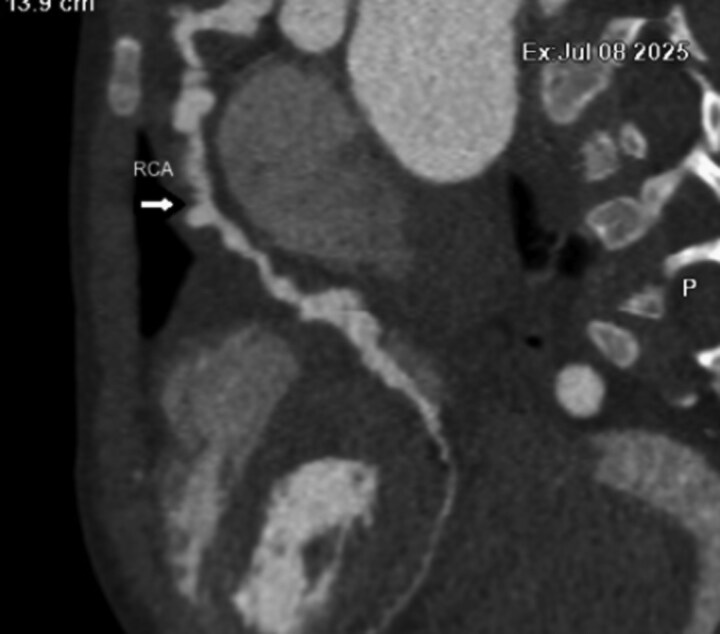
Computed tomography coronary angiography showing multiple aneurysms in the right coronary artery (RCA). The arrow indicates the RCA aneurysms.
